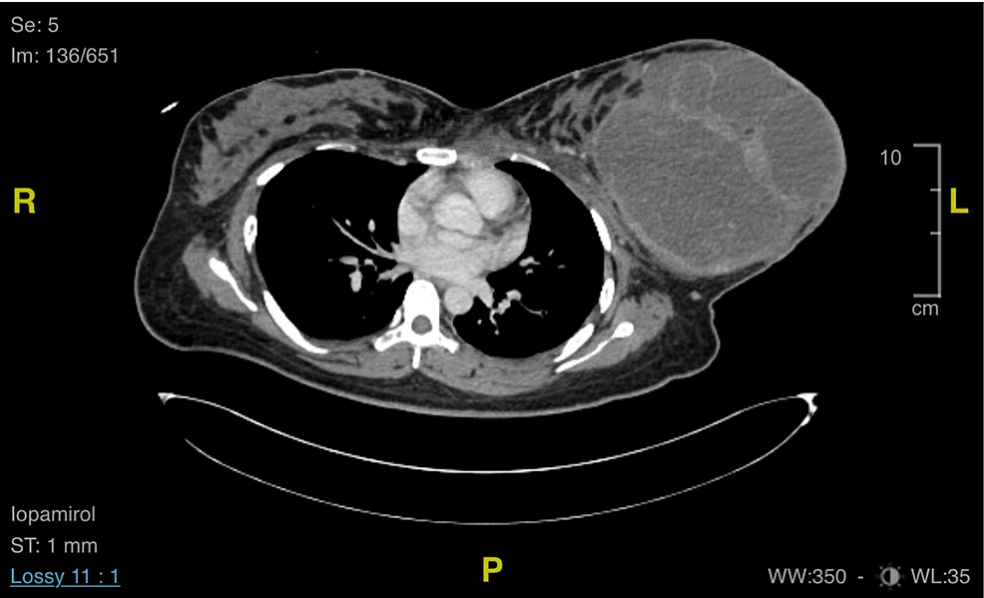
CT TAP shows a large heterogeneous left breast mass which is mainly cystic in nature with the presence of thick enhancing septations, surrounded by a thick, irregular enhancing wall with surrounding fat streakiness and nodal metastasis. CT TAP: Computed Tomography (CT) Thorax, Abdomen and Pelvis
